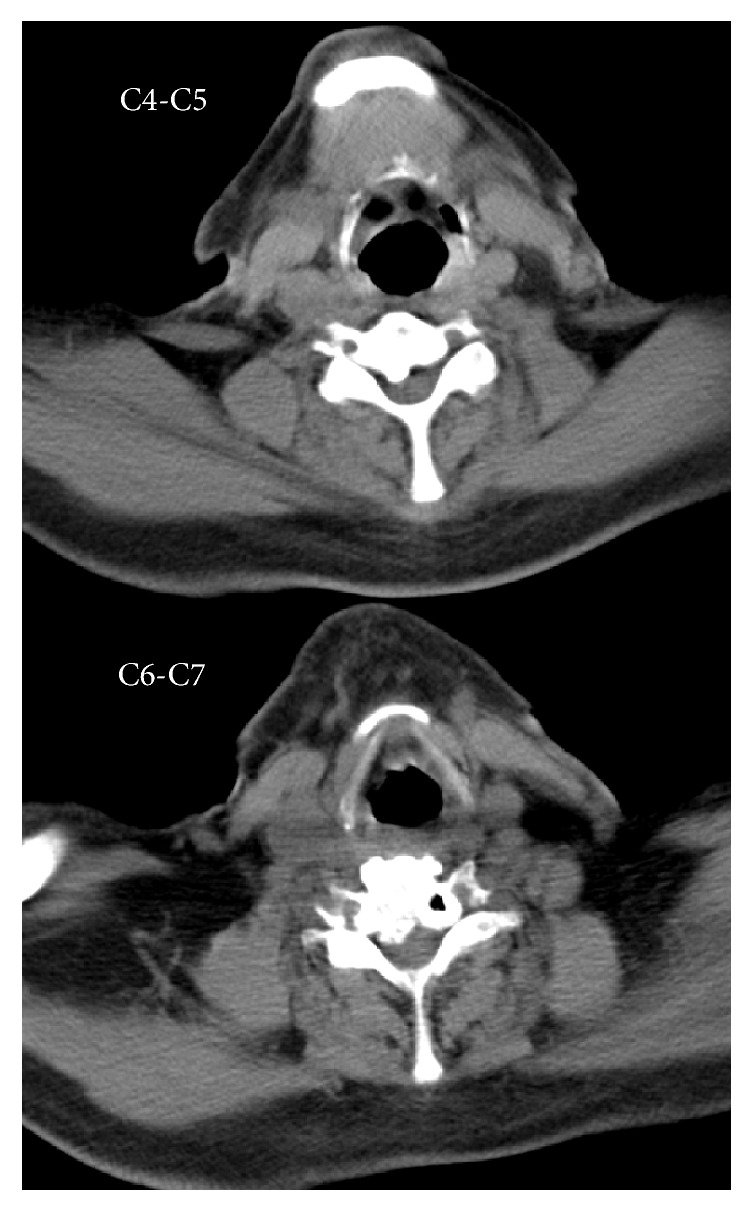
Neck CT scan C4-C5, C6-C7.
